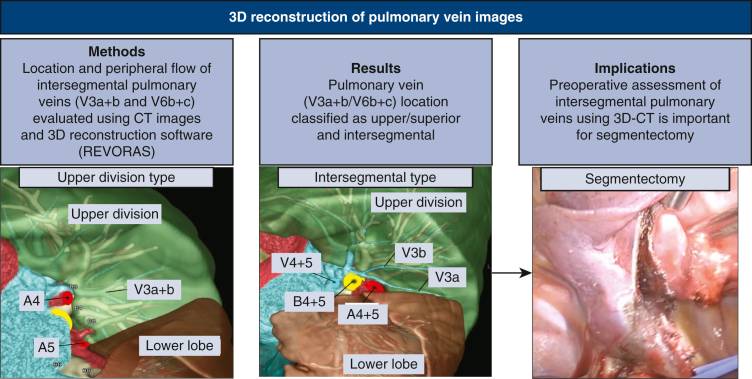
Preoperative evaluation of intersegmental pulmonary veins for segmentectomy with volume-rendering 3-dimensional computed tomography (*3D-CT*) image reconstruction.
